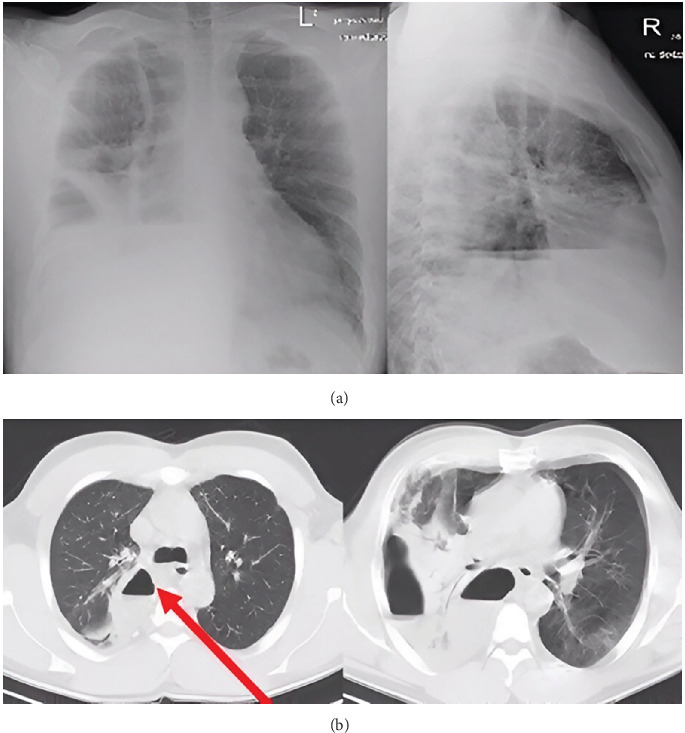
(a) Chest x-rays and (b) CT scans performed on the day of the patient's admission to the hospital (1st of February 2024)—Case 1. (b) The abscess wall is marked with an arrow.
